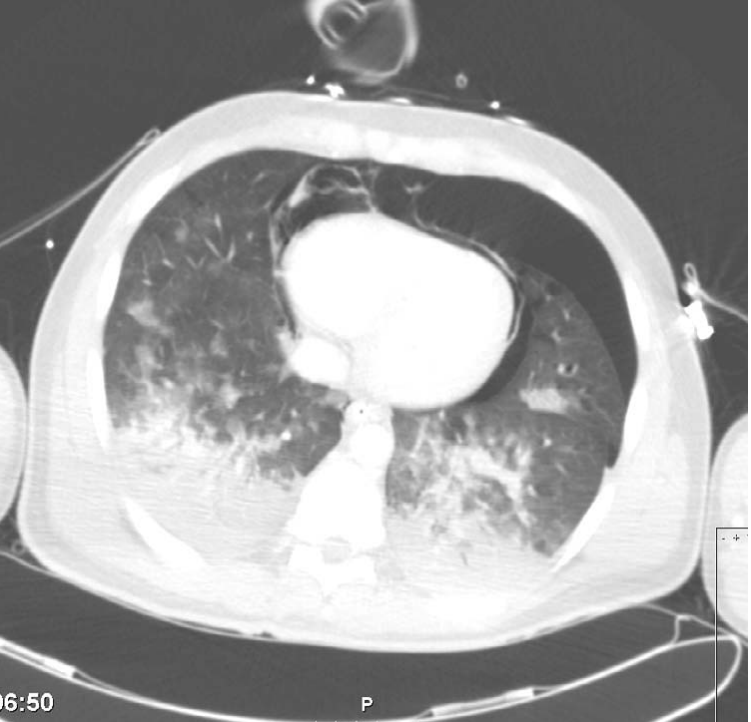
CT scan, transversal view, showing pneumothorax, pneumomediastinum and pneumopericardium. Haematothorax is present at the time of scanning.
